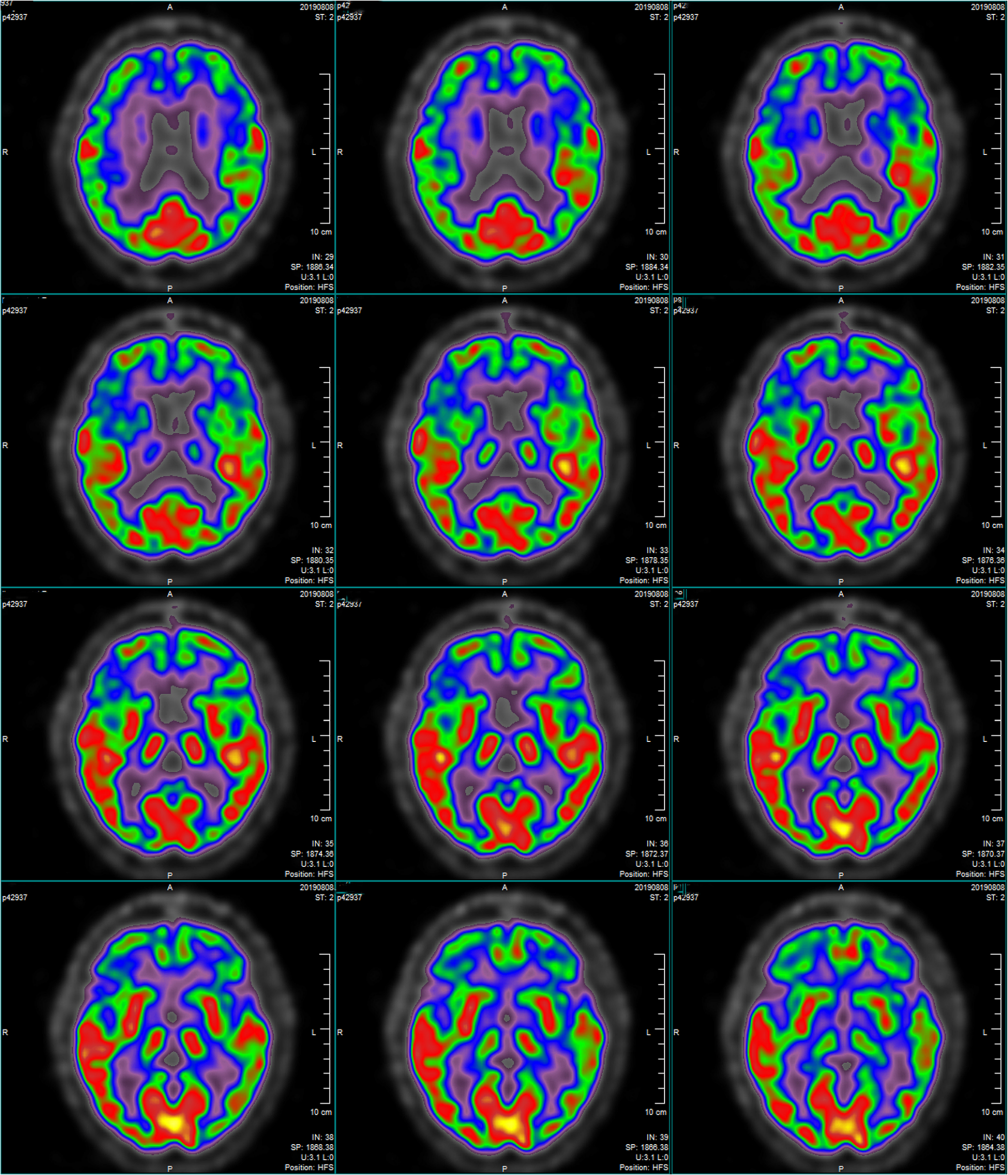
The 18F-FDG PET/CT in August 2019. Extensive areas of hypometabolism in frontal cortex and head of caudate nucleus
